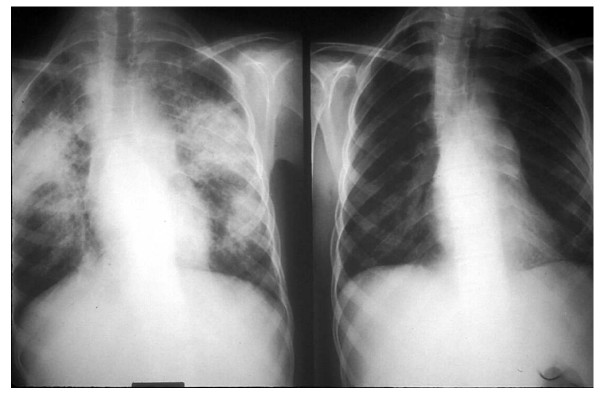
Pulmonary infiltrates of sarcoidosis: Chest radiograph in a 12-year-old female with sarcoidosis showing patchy, diffuse alveolar infiltrates involving both lungs (Left). A repeated study 6 months later with significant improvement (Right).
